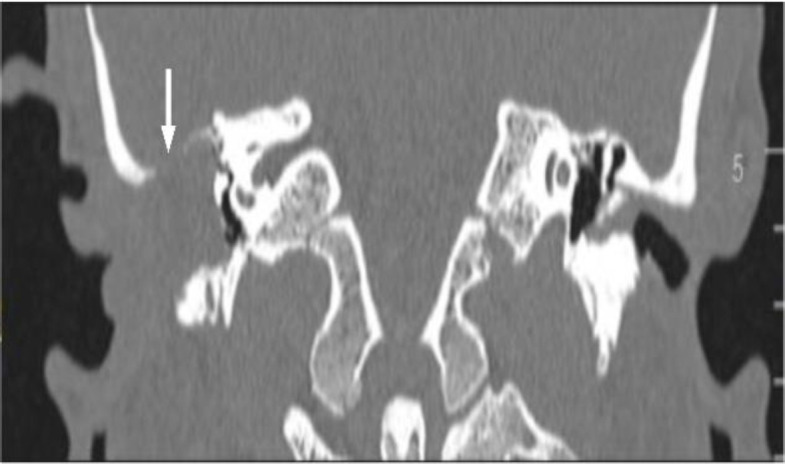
CT scan. Coronal view. A right skull base defect is observed
